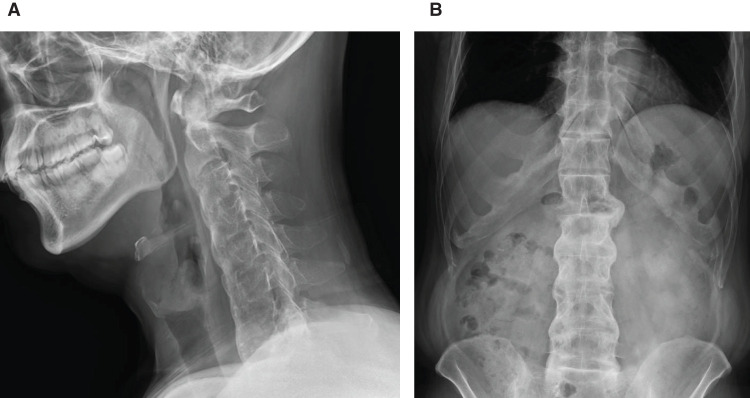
Lateral plain X-ray of the cervical spine (A) and anteroposterior plain X-ray of the abdomen (B). The cervical and lumbar vertebrae show fusion and deformity resembling bamboo nodes, demonstrating findings consistent with the bamboo spine.
